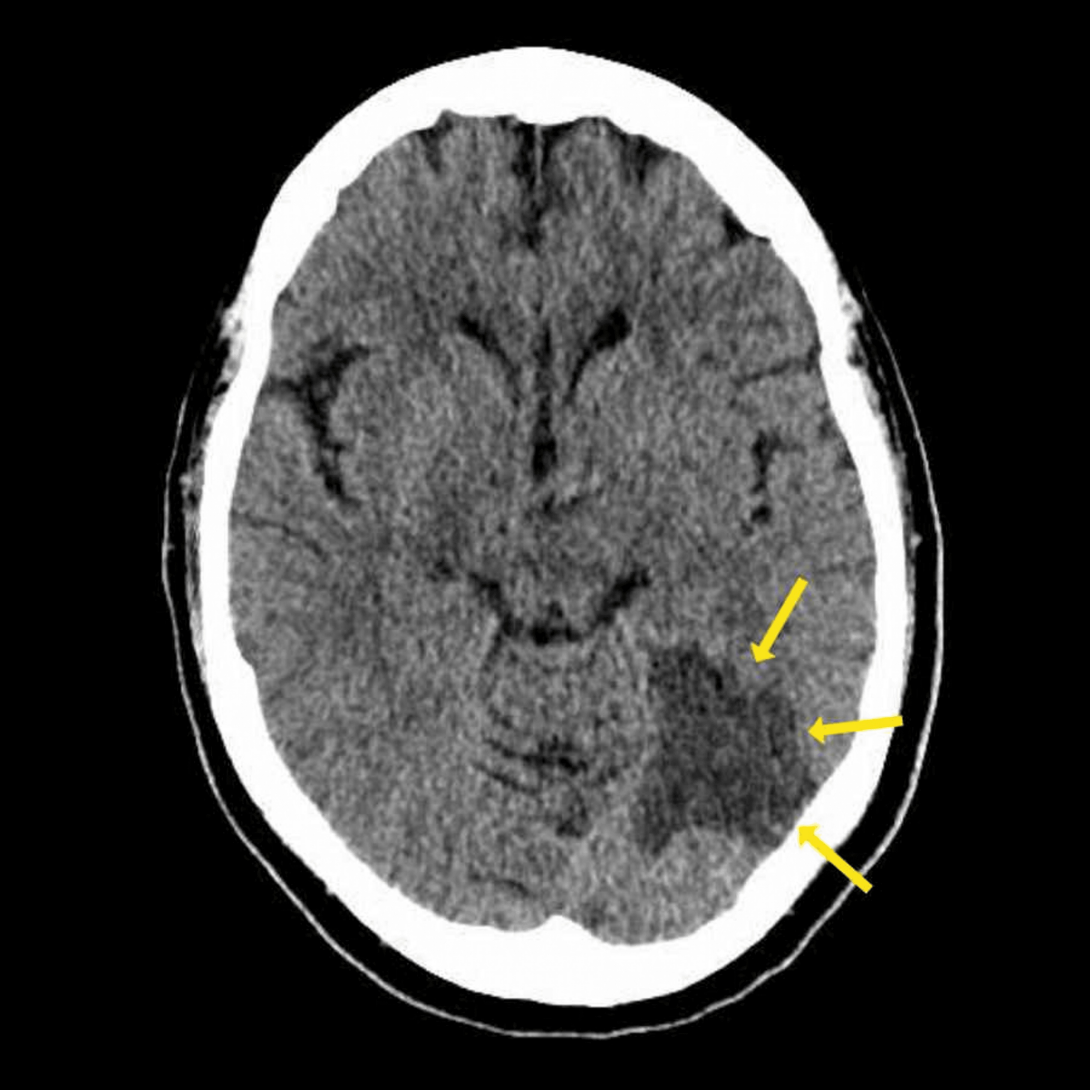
CT Head Without Contrast CT image showing loss of normal grey-white matter differentiation and hypoattenuation in the left posterior and medial temporal lobe (yellow arrows, with regional sulcal effacement, consistent with an acute or subacute infarction in left PCA territory. PCA: posterior cerebral artery
